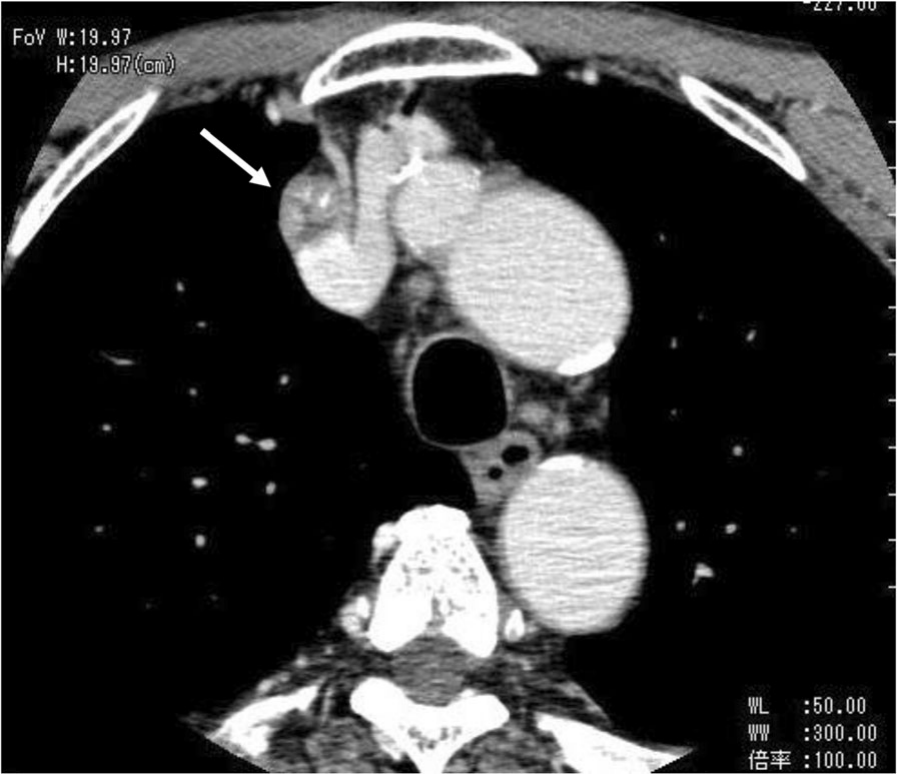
Computed tomography shows enlargement of the solitary superior mediastinal lymph node, and invasion to the right brachiocephalic vein was suspected *(arrow)*
